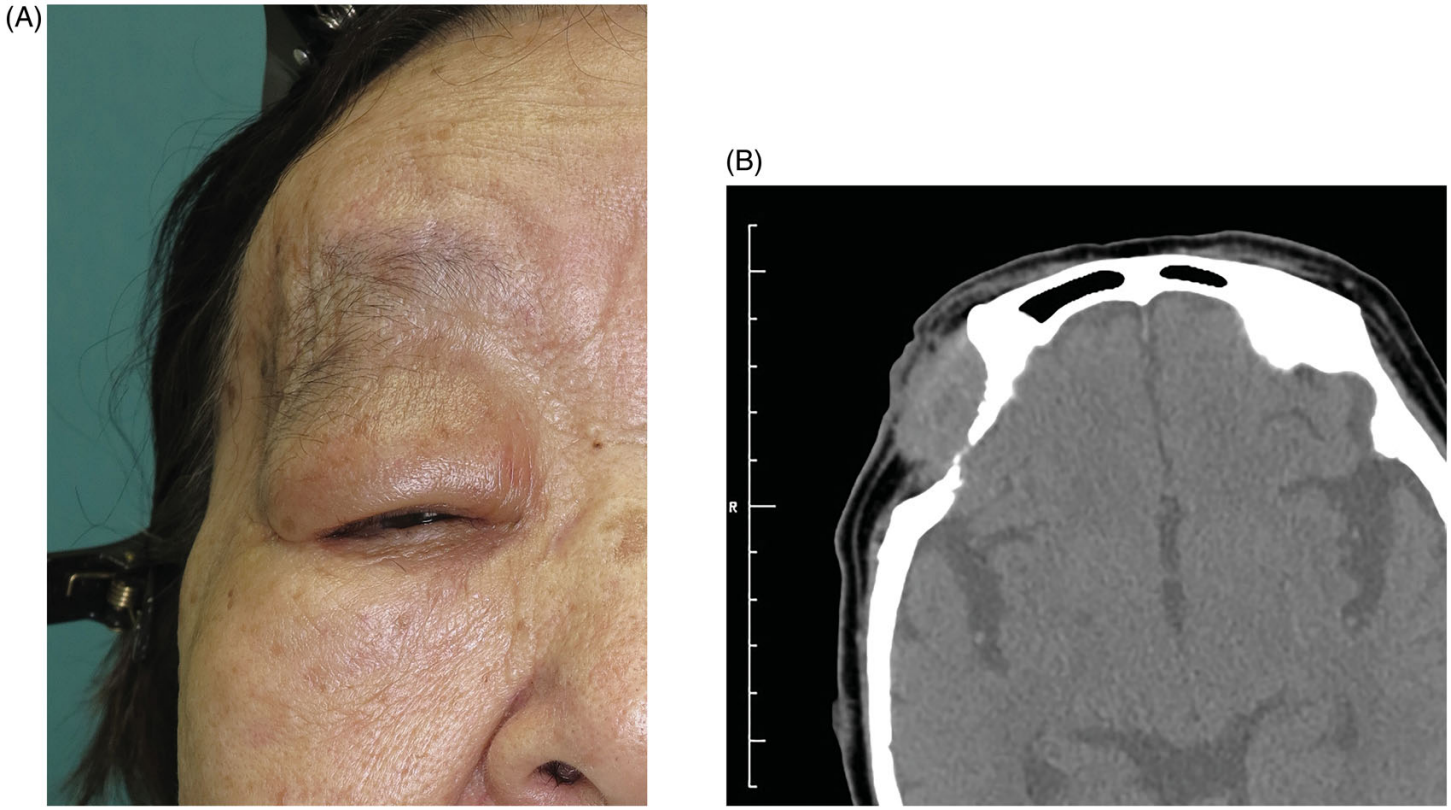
Gross appearance (A) and computed tomography (B) at 1 year after final sclerotherapy. The cyst did not disappear, but shrank to become inconspicuous in appearance.
